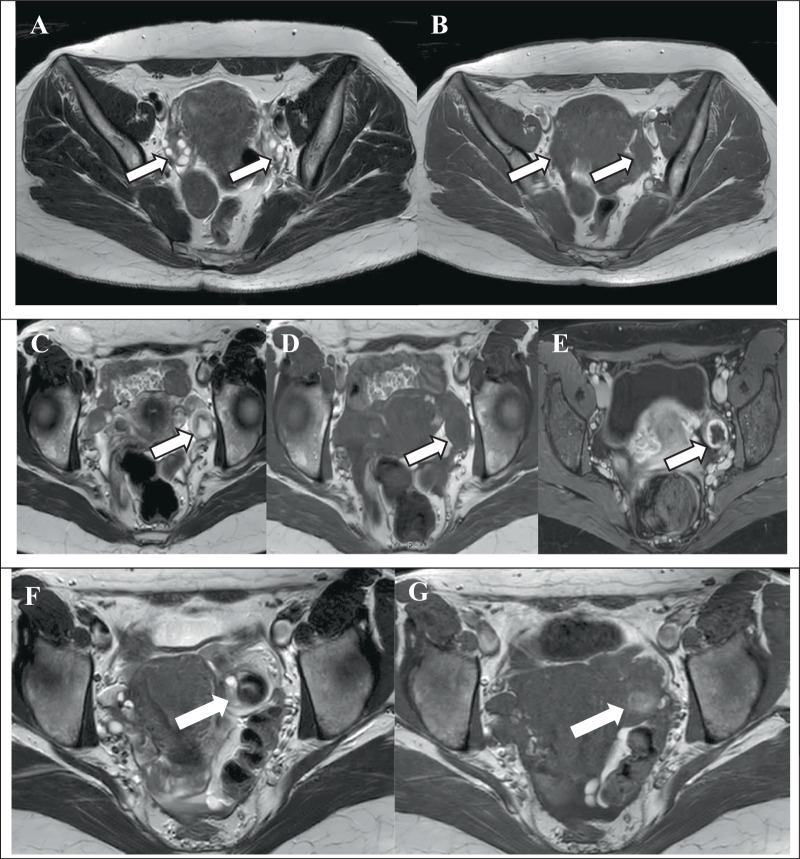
O-RADS MRI 1: cystic physiological ovarian lesions. Ovarian follicles: axial T2-weighted (A) image shows hyperintense follicles (white arrows), and axial T1-weighted image (B) shows hypointense follicles of both ovaries (white arrows). Corpus luteum cyst: axial T2-weighted image (T2WI) (C) and axial T1-weighted image (D) showing the cyst with a thickened wall (white arrow). Post-contrast T1-weighted fat-suppressed (T1Wfs) (E) image demonstrating peripheral rim enhancement of the cyst (white arrow). Haemorrhagic ovarian cysts: axial T2WI (F) shows a haemorrhagic ovarian cyst with dense internal fluid (white arrow), while axial T1-weighted image (G) demonstrates a hyperintense signal of the cyst due to haemorrhagic content (white arrow)
